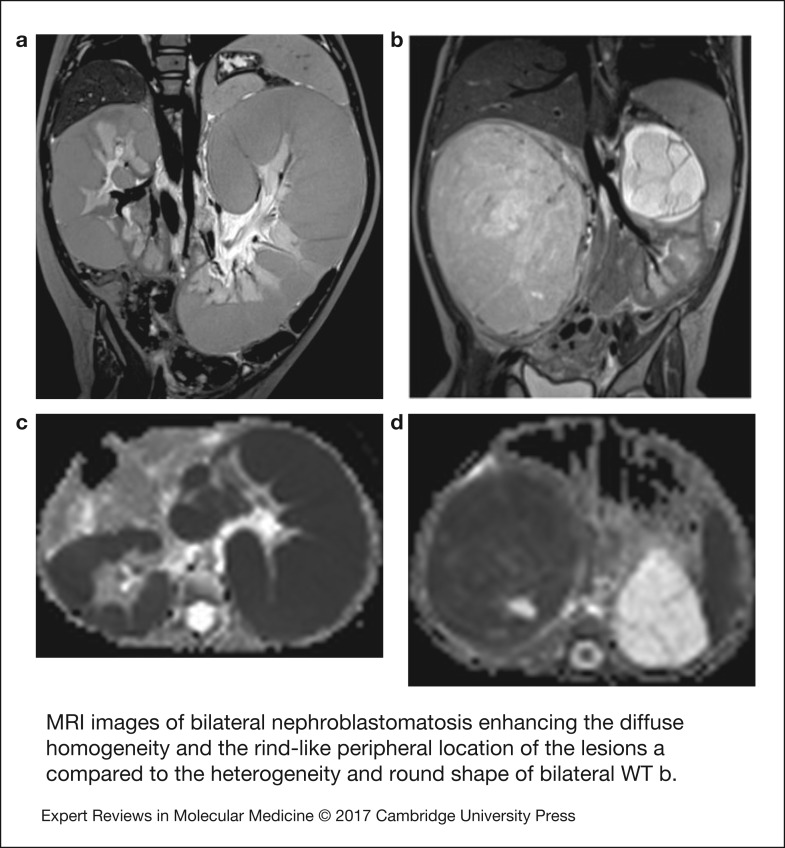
MRI images of bilateral nephroblastomatosis enhancing the diffuse homogeneity and the rind-like peripheral location of the lesions (a) compared with the heterogeneity and round shape of bilateral WT (b). The left kidney seems suitable to a nephron sparing surgery as the mass arises from the superior pole above the left pedicle (b). Corresponding apparent diffusion coefficient (ADC) maps demonstrate low ADC in both kidneys with nephroblastomatosis (c) but different ADC values between the right solid nephroblastoma and the left cystic tumour (d).
